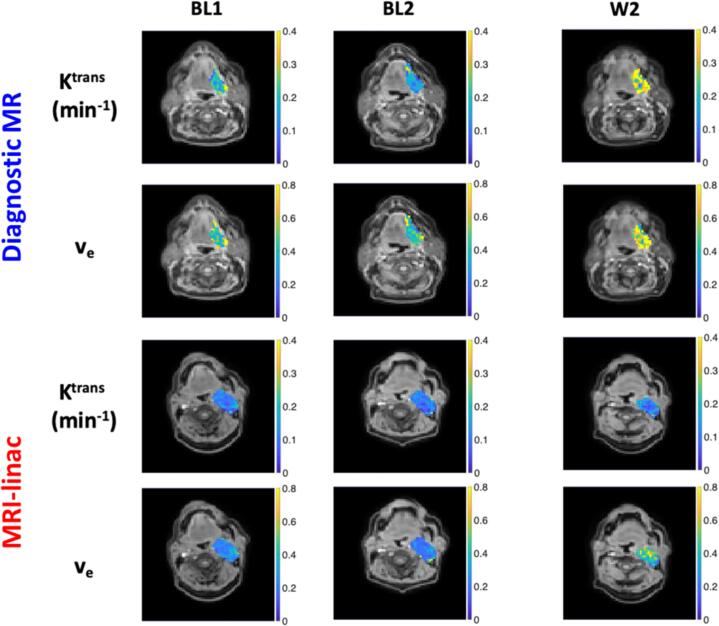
Example primary tumour K^trans^ and v_e_ parameter maps overlaid onto the FFE (⍺ = 5°) image for two patients acquired on the diagnostic MR and MRI-linac systems at baseline timepoints (BL1, BL2) and week 2 (W2) of treatment. Example IAUC_60_ and T_1_ maps are provided for the same lesions in.
